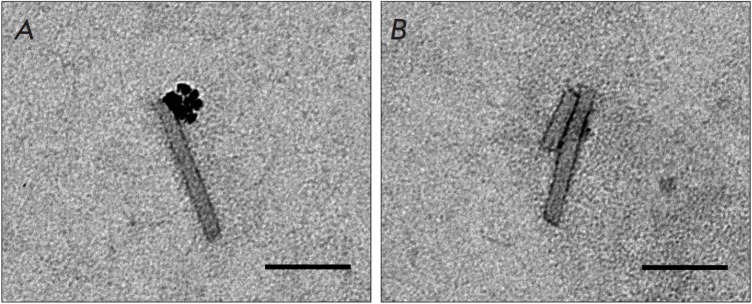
MNPs form complexes with one end of ultrasound-treated TMV (TMV^US^).
